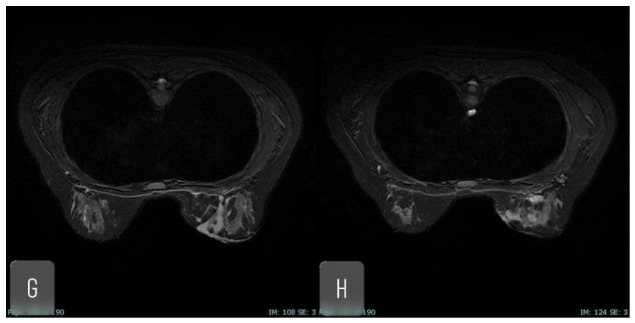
Thirty-five yo. woman, 2 weeks postpartum, with clinical picture compatible with lactational mastitis. No significant abscesses detectable with ultrasound. Ultrasound detects tubular pattern. An MRI was performed, which detected multiple coalescent contrast enhanced areas; no abscesses were found. A biopsy was performed which detected chronic phlogistic lymphoplasmacellular infiltrate. (A–C) US imaging, no abscesses were found; tubular pattern is detectable; (D) MRI, MIP; (E,F) MRI, Contrast enhanced imaging; (G,H) MRI, T1 imaging.
